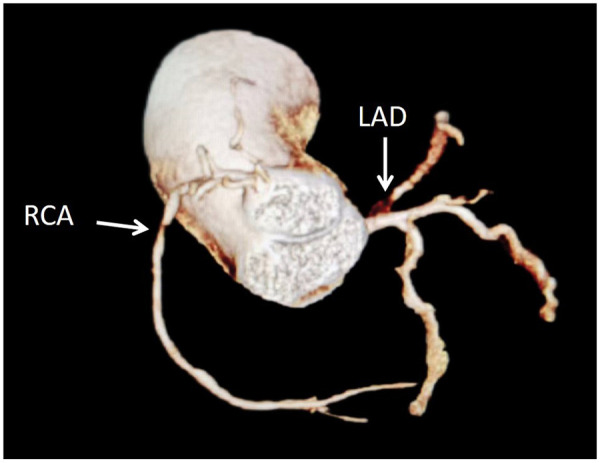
Pre-procedural coronary computed tomography angiography (CTA) serving as the sole anatomical roadmap. The CTA, obtained from an external hospital, shows severe stenosis in the proximal right coronary artery (RCA, arrow) and significant disease involving the left main (LM) and left anterior descending (LAD) artery bifurcation (arrowhead). This image served as the only pre-procedural roadmap for the zero-contrast PCI.
